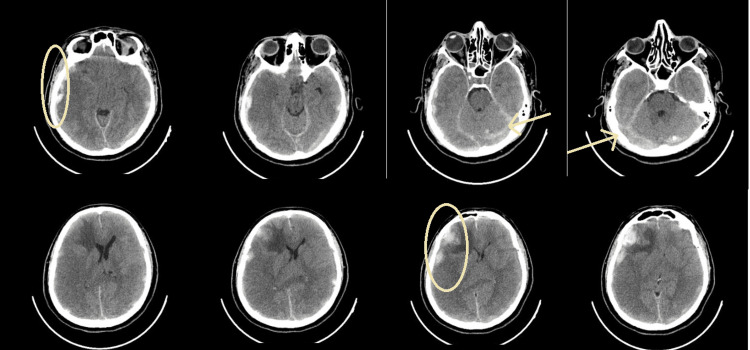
Complex vascular abnormalities in brain CT scan, suggestive of venous thrombosis, presenting with multiple subdural and intracerebral hematomas in the right frontal and left parietal lobes.
